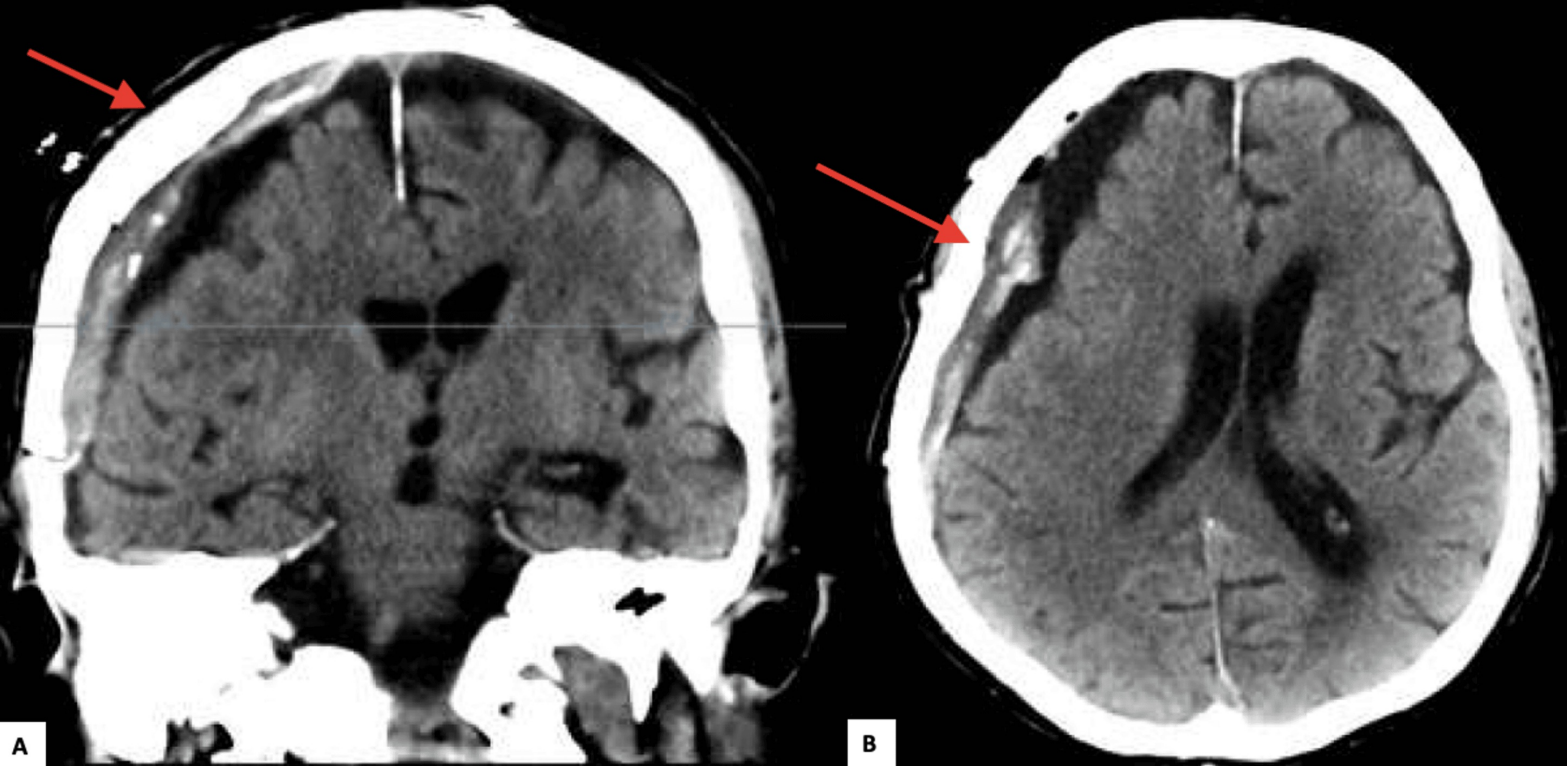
Post-cranioplasty CT scan showing no signs of infarction and no new intracranial bleeding (A: coronal; B: axial). The blue line in the coronal section represents the axial cut section accordingly.
